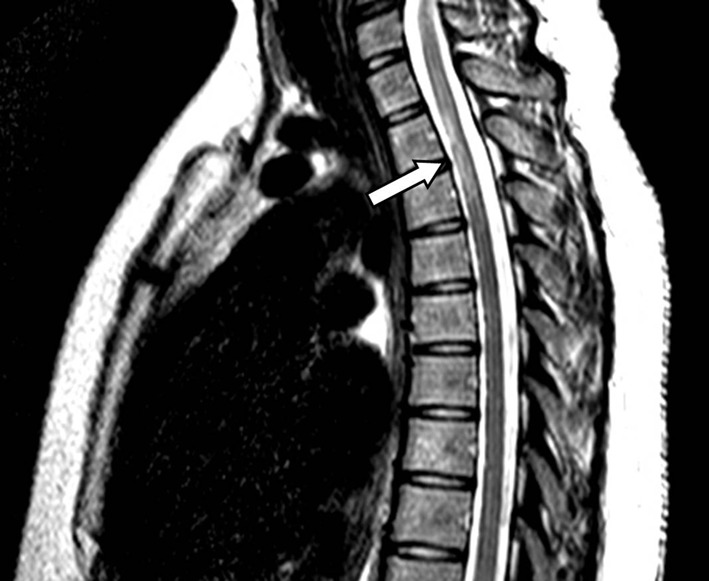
Sagittal T2-weighted MRI of the spine reveals a hyperintense lesion (arrow) at the T1-T3 levels of the spinal cord.
